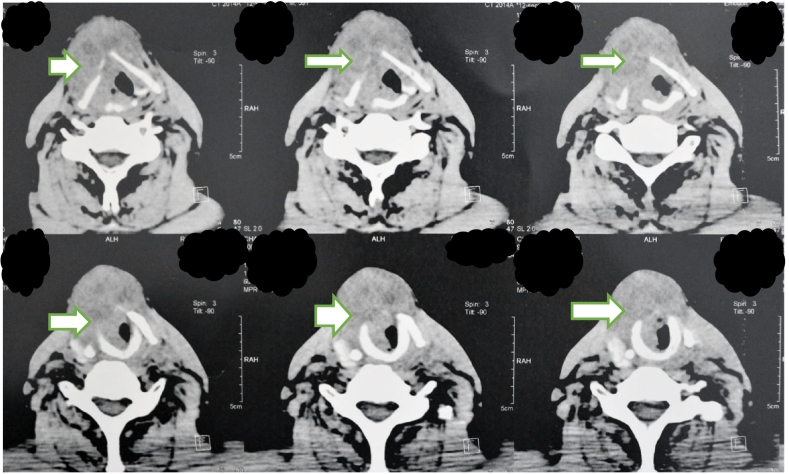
Cervical CT scan showing a laryngeal tumor occupying all three laryngeal stages.
